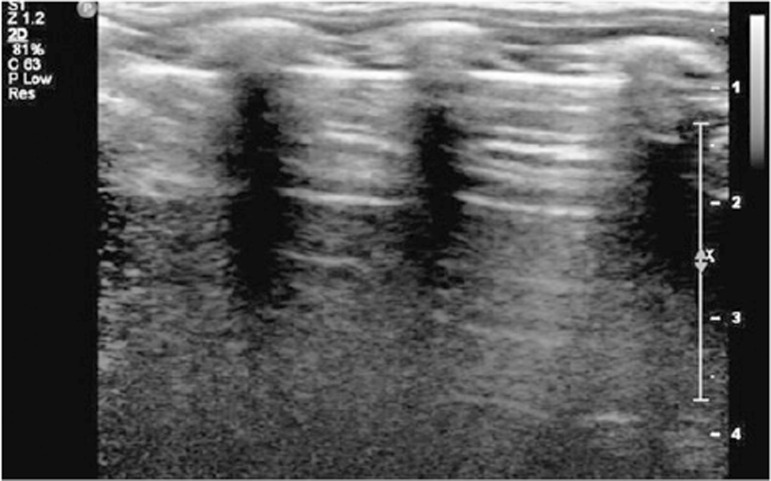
Ultrasonographic A-line artifacts: horizontal lines equidistant and parallel to the pleural line
